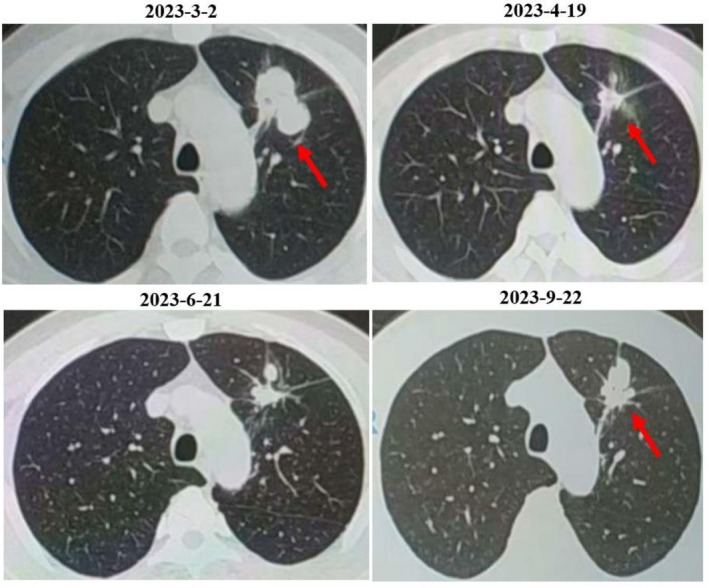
Selected axial chest CT images (lung window) showing interval changes in the primary lung lesion during late almonertinib monotherapy and subsequent almonertinib plus savolitinib treatment. The lesion (red arrows) initially decreased, then regrew at progression, and decreased again after addition of savolitinib.
